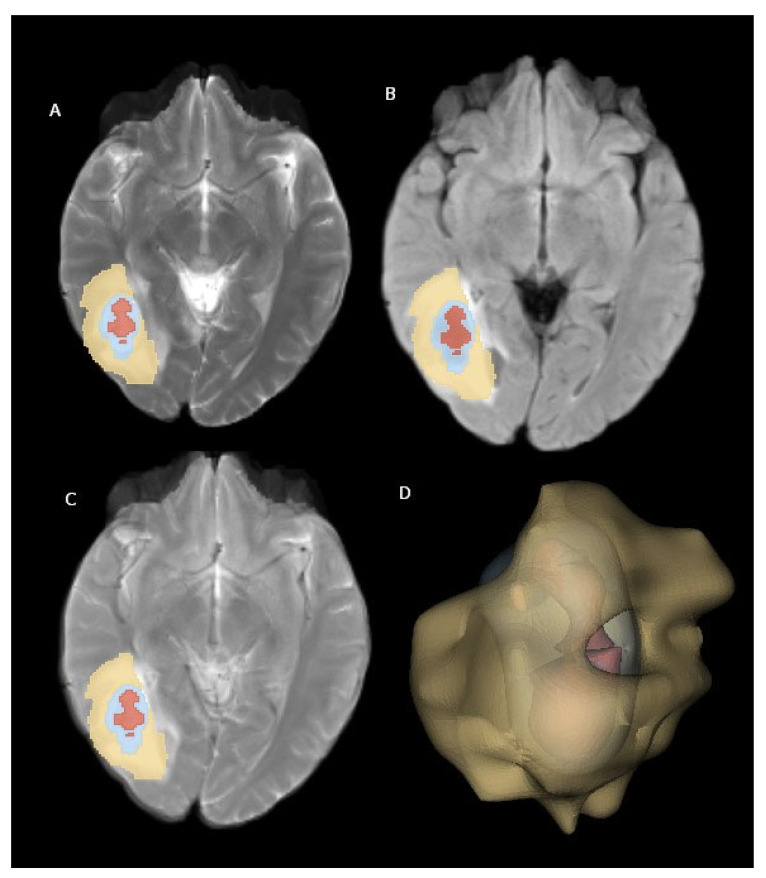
Brain metastasis from NSCLC located in right cerebral hemisphere. (A) T2-weighted images, (B) FLAIR-weighted images, (C) T2-FLAIR digital subtraction images, and (D) three-dimensional volume rendering of mass. Yellow: perilesional edema area; blue: contrast-enhanced area; red: necrosis area.
